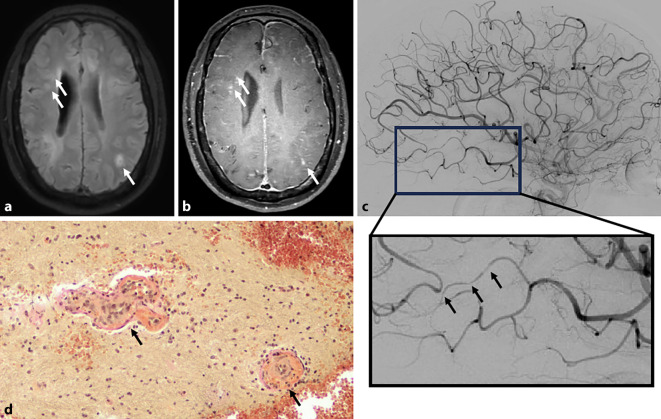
Charakteristische Befunde bei Patienten mit primärer Angiitis des zentralen Nervensystems (PACNS). a Magnetresonanztomographie (MRT) mit FLAIR(„fluid attenuated inversion recovery“)-Sequenz, die multiple demyelinisierende Läsionen aufzeigt. b MRT unter Verwendung von Black-blood-Sequenzen, welche einerseits eine teilweise deutliche Kontrastmittelaufnahme der Läsionen demonstrieren (*Pfeile*) und andererseits eine ausgeprägte Kontrastmittelanreicherung der Pachy- und Leptomeningen zeigen, als Indikator der floriden Entzündung. c Digitale Subtraktionsangiographie (DSA), die multiple Kaliberschwankungen aufweist, exemplarisch im vergrößerten Bildausschnitt durch *Pfeile* markiert. d Elastica-van-Gieson(EvG)-Färbung eines Biopsats mit durch *Pfeile* gekennzeichneten Blutgefäßen, die Gefäßwandnekrose und einzelne Lymphozyten in der Gefäßwand zeigen (Vergr.: 200-fach)
